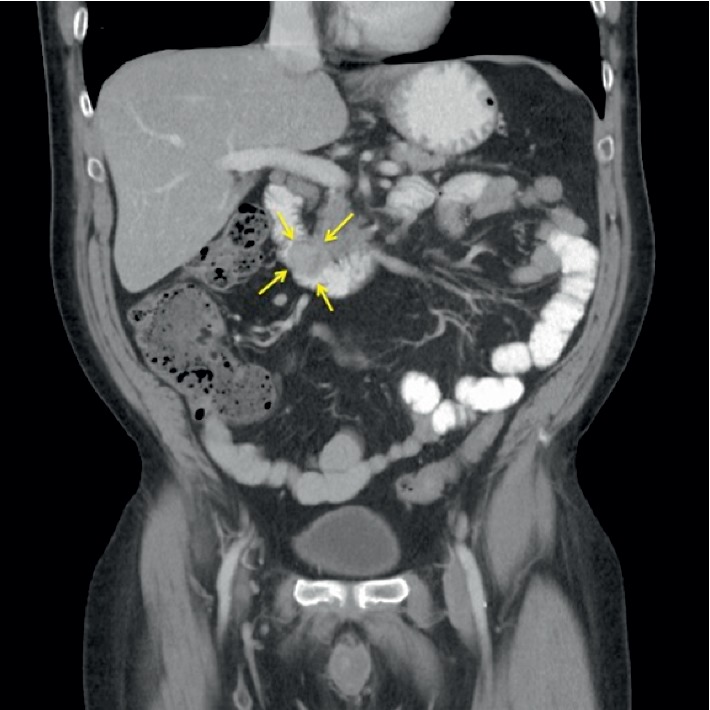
CT imaging showing a 2.6 × 1.4 cm intraluminal mass involving the proximal duodenum extending to the level of the ampulla.
